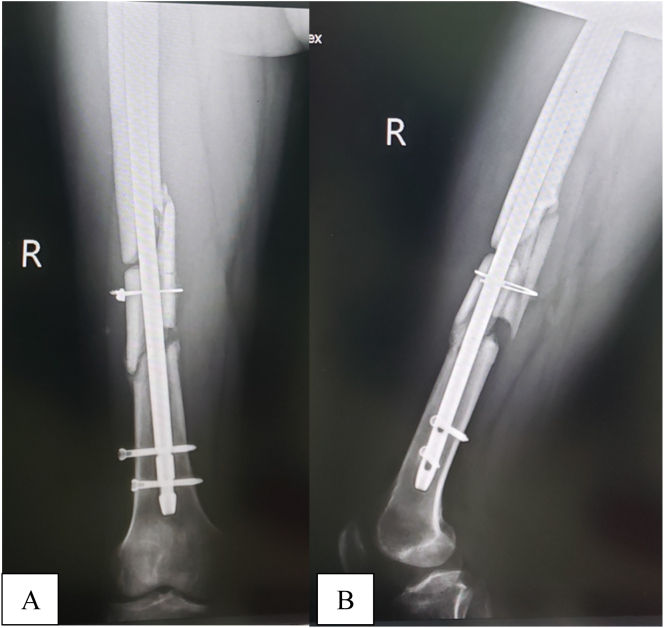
Immediate postoperative radiographs of the femur. Anteroposterior (A) and lateral (B) views showing a locked intramedullary femoral nail. Note the cerclage cable stabilising the segmental fracture.
